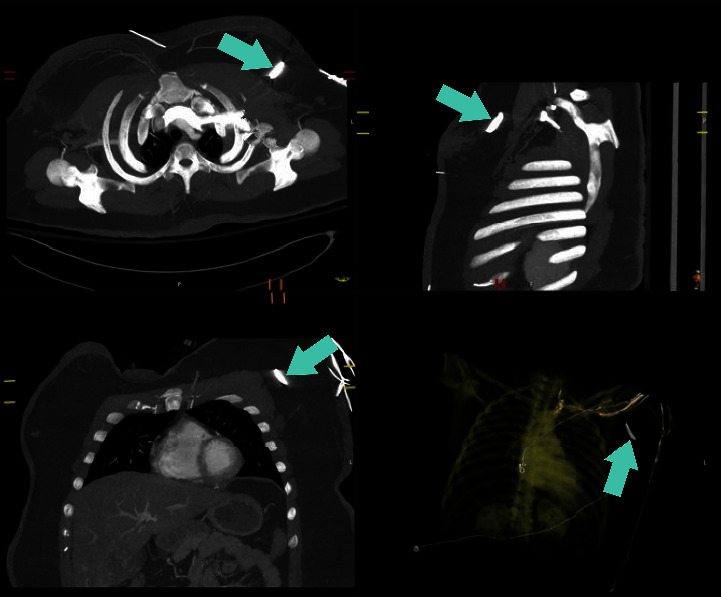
CT angiogram demonstrating the metallic foreign body.
